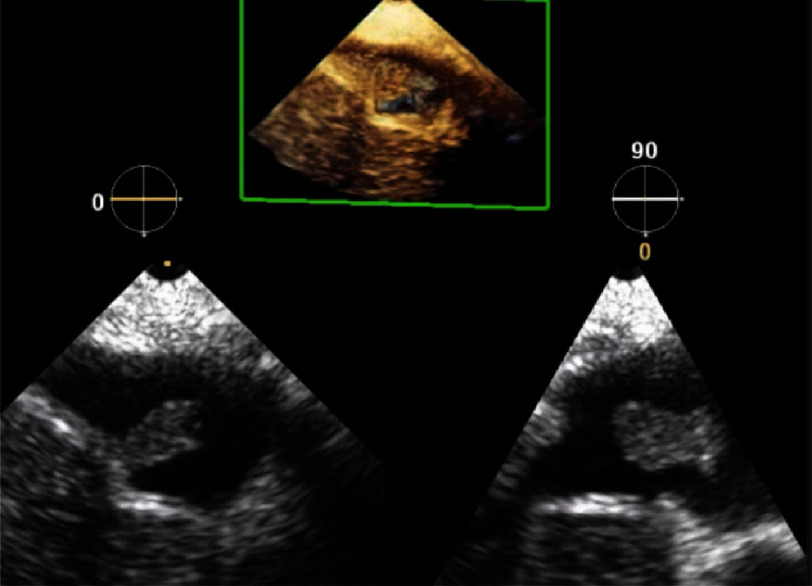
Intraoperative transesophageal echocardiography and 3D image of the amniotic fluid embolism.
